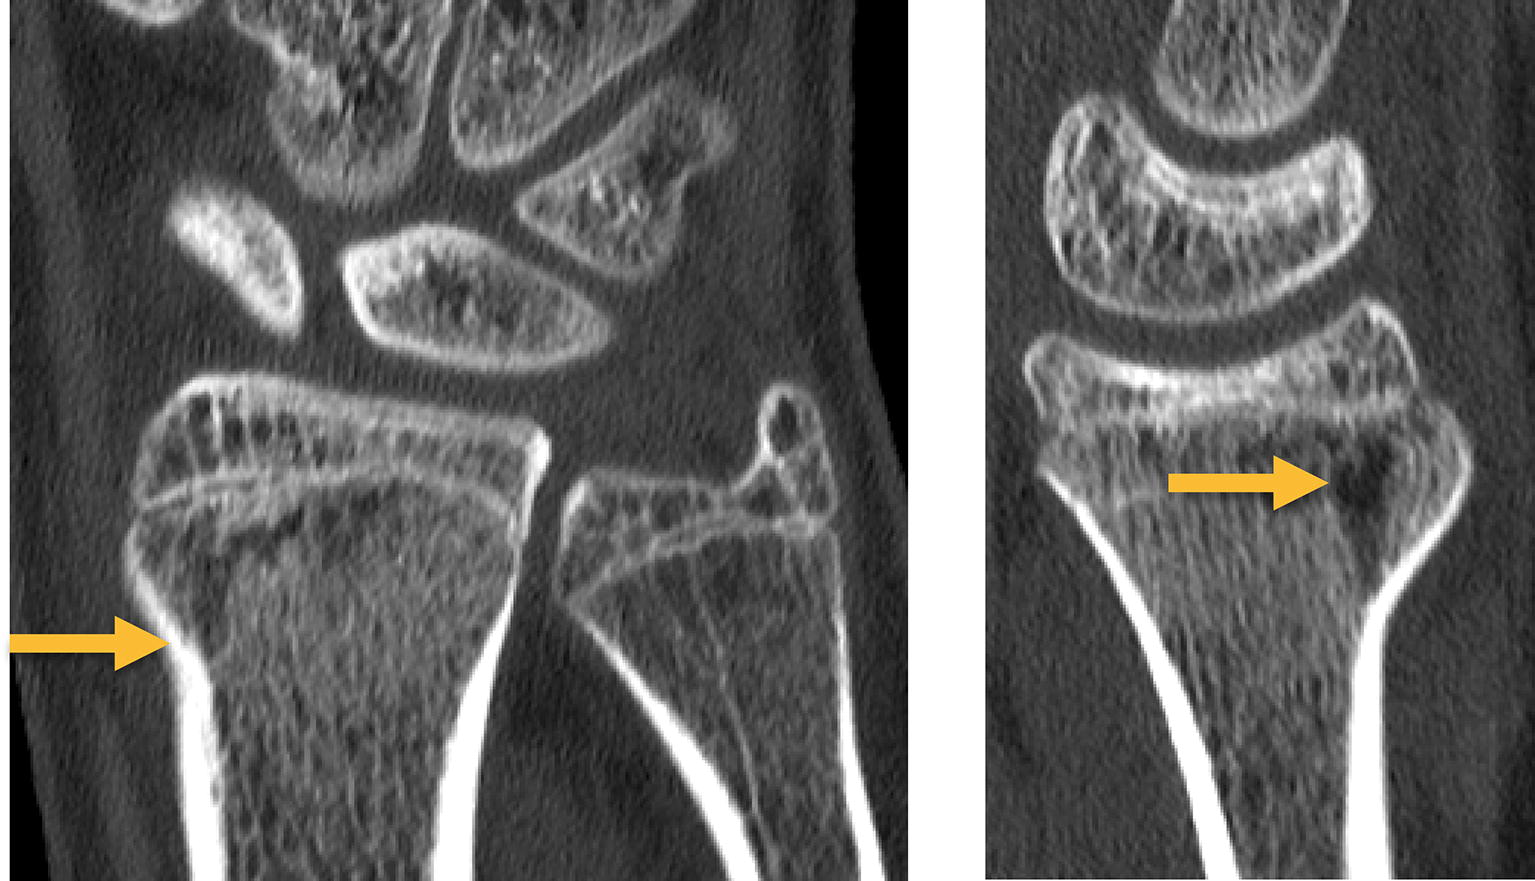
Diagnostic imaging case 3. CT scan demonstrating a minimal displaced transitional fracture of the right distal radius (arrow)
